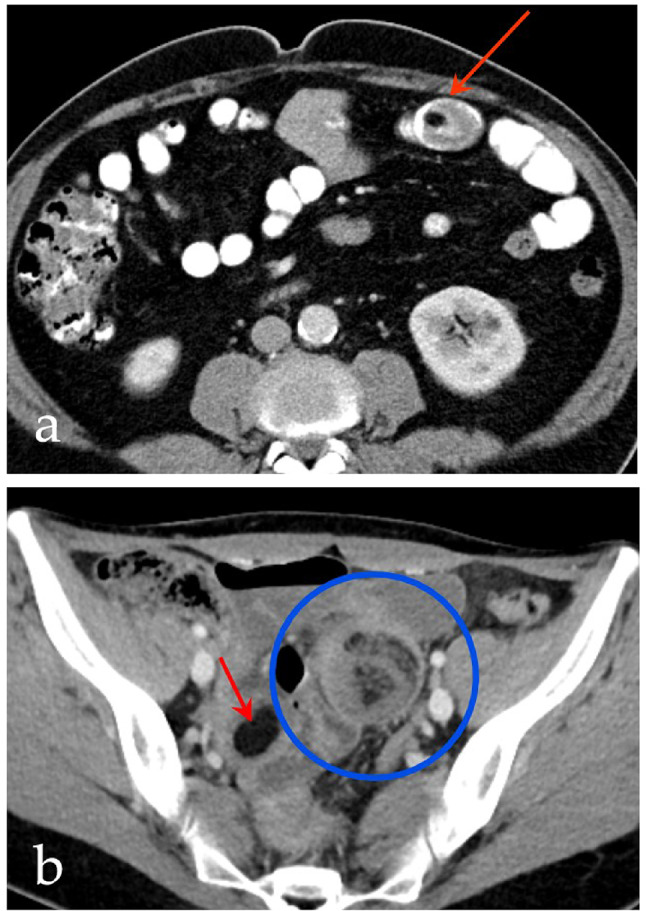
Axial contrast-enhanced CT images demonstrating adult intussusceptions caused by a benign pathological lead point. The red arrows in both panels (a, b) point to well-defined, fat-attenuating masses consistent with enteric lipomas acting as the lead points. The blue circle in panel (b) highlights the edematous, multi-layered bowel loops characteristic of the intussusception
